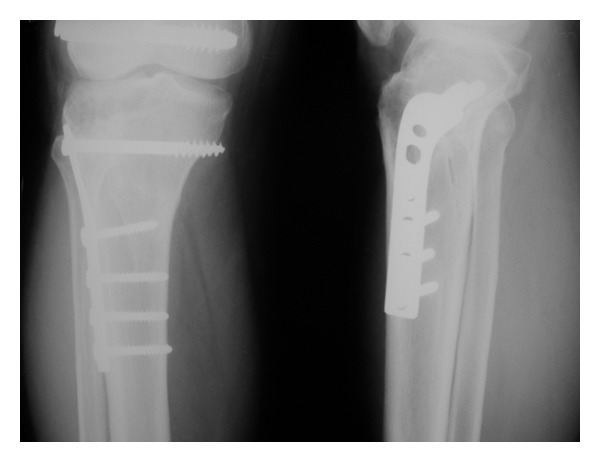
X-ray right leg AP and lateral views at 2-year followup showing fracture union.
